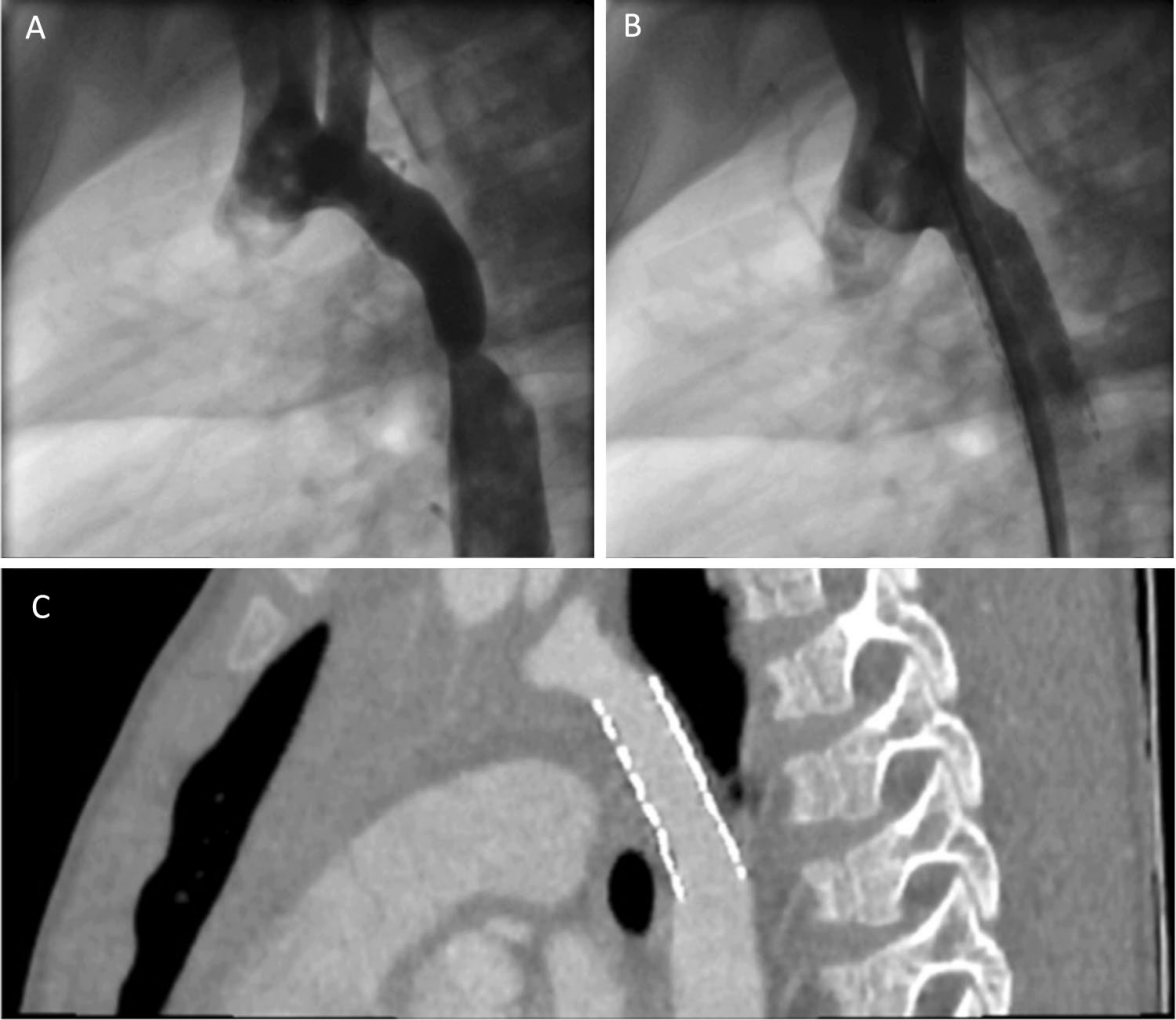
Aortograms and CT of case 17 using Formula 535 stent. A Lateral aortogram demonstrating the descending thoracic CoA. B Lateral aortogram demonstrating a well-expanded 9 mm × 30 mm stent in position with vessel calibre matching. C CT performed 3 months post stent demonstrating no aortic wall complications or stent fractures
